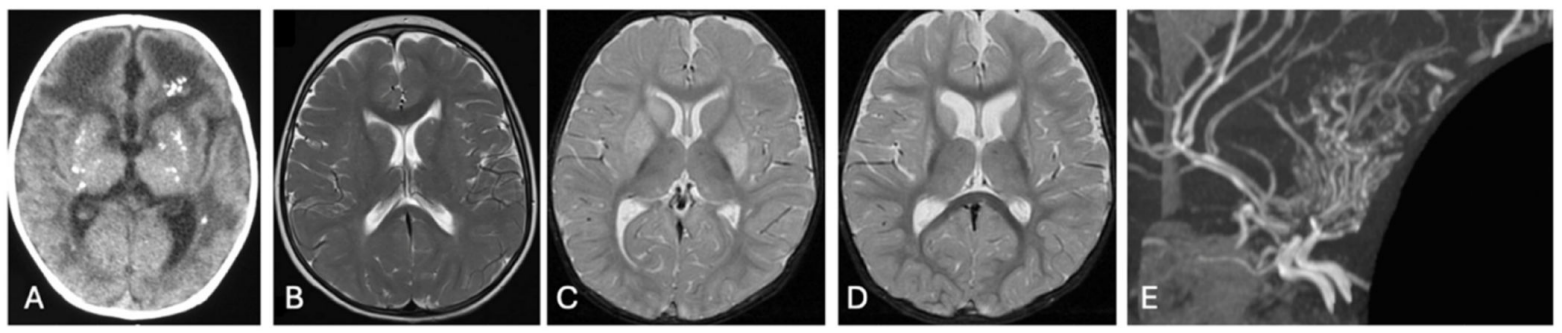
Characteristic imaging features seen in type I interferonopathies A. Axial cranial CT at age 1 year of a child with biallelic loss-of-function mutations in TREX1 showing characteristic calcification of the basal ganglia and extensive white matter abnormalities particularly frontally. B. Cranial axial T2 MRI at age 6 years of a child with a heterozygous gain-of-function mutation in IFIH1 with severe four-limb spasticity but only minimal posterior periventricular white matter high signal abnormalities. C. Cranial axial T2 MRI at age 8 months of a child with biallelic loss-of-function mutations in ADAR1 showing high signal change in the basal ganglia bilaterally. D. Same child as in C at age 2 years 11 months showing marked shrinkage of the basal ganglia. E. MRA (lateral view) at age 7 years of a child with biallelic loss-of-function mutations in SAMHD1 showing stenoses of terminal internal carotid and basilar arteries with extensive collateral formation (moyamoya).
